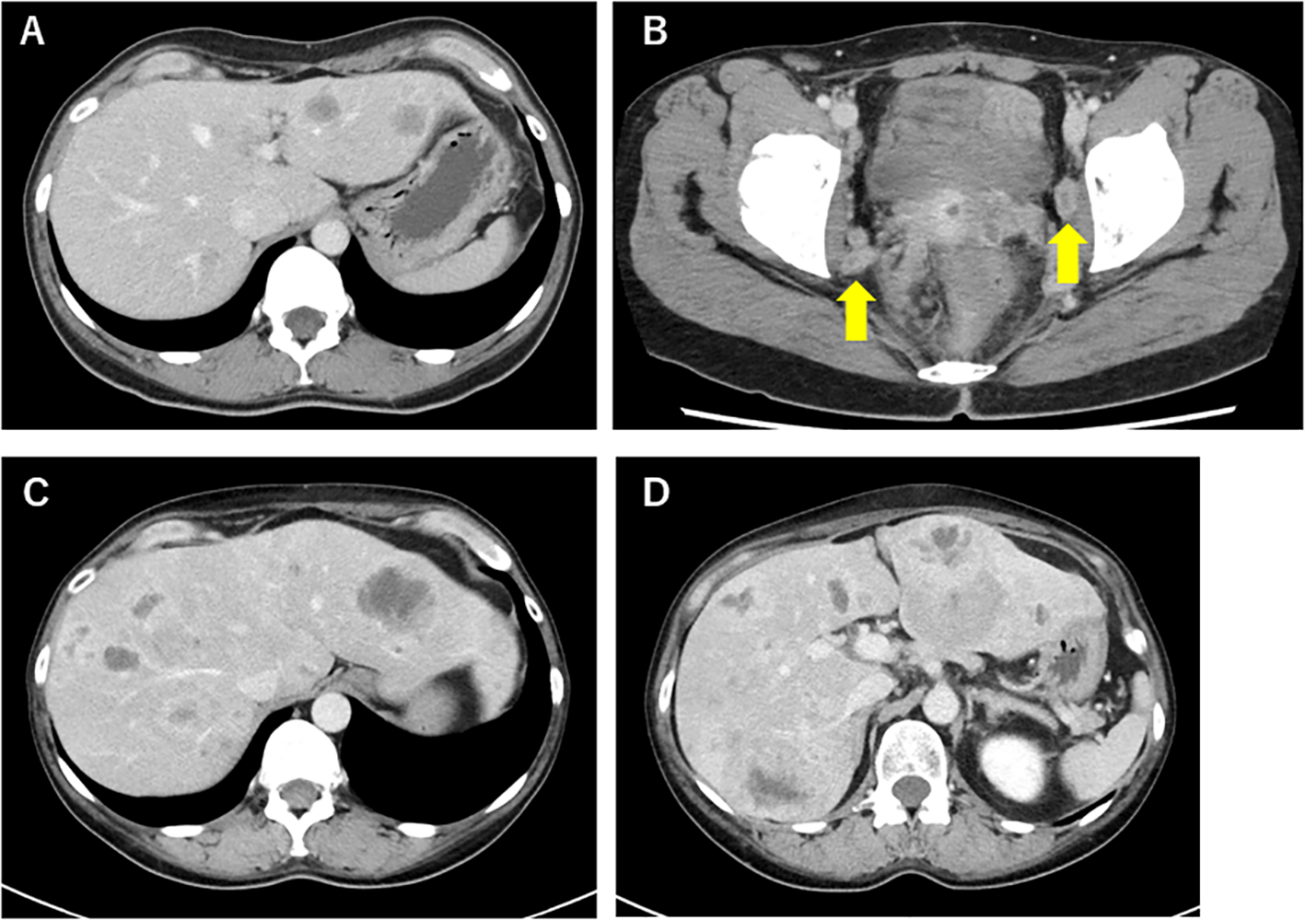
Abdomen CT scan 5 months after her first chemotherapy, which revealed multiple metastases of the liver (A) and lymph nodes (B). Abdomen CT scan for psychiatric symptoms (Day 1). This revealed an increase in the size of liver metastases (C, D).
